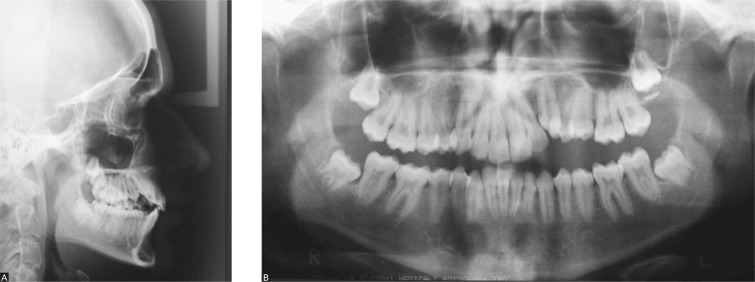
Pretreatment radiographs. A) Cephalometric radiograph; B) panoramic
